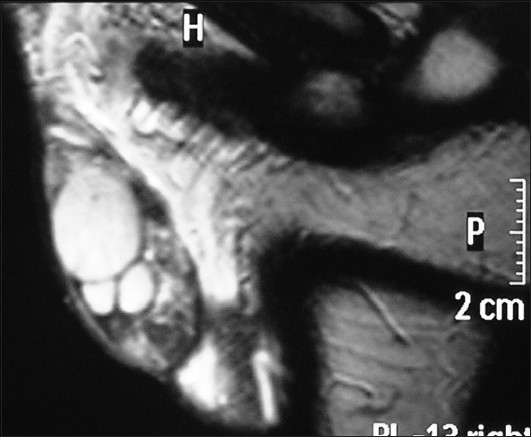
Sagittal T2-weighted scan of the right hemiscrotum with supernumerary testis at the lower pole
